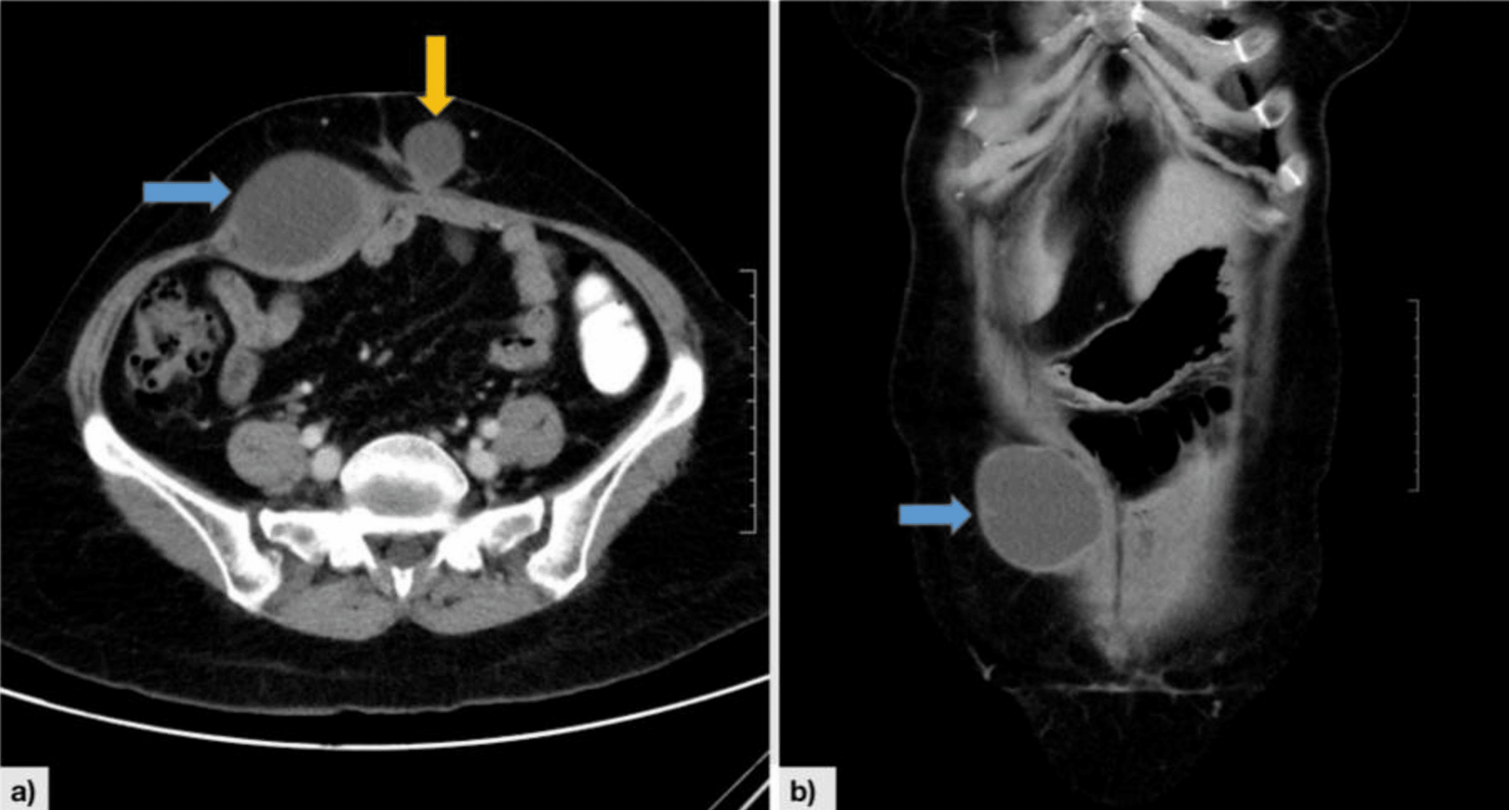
This contrast-enhanced computed tomography of the abdomen and pelvis image shows recurrent lesions (second time) in the form of two hypodense cystic lesions in the axial (a) and coronal (b) sections. One is located in the anterior abdominal wall in the right rectus abdominis muscle, measuring 5.3 x 7.3 cm and indicated by a blue arrow. The other lesion is in the subcutaneous plane along the left paramedian aspect of the midline, measuring 2.5 x 2.8 cm and indicated by a yellow arrow.
